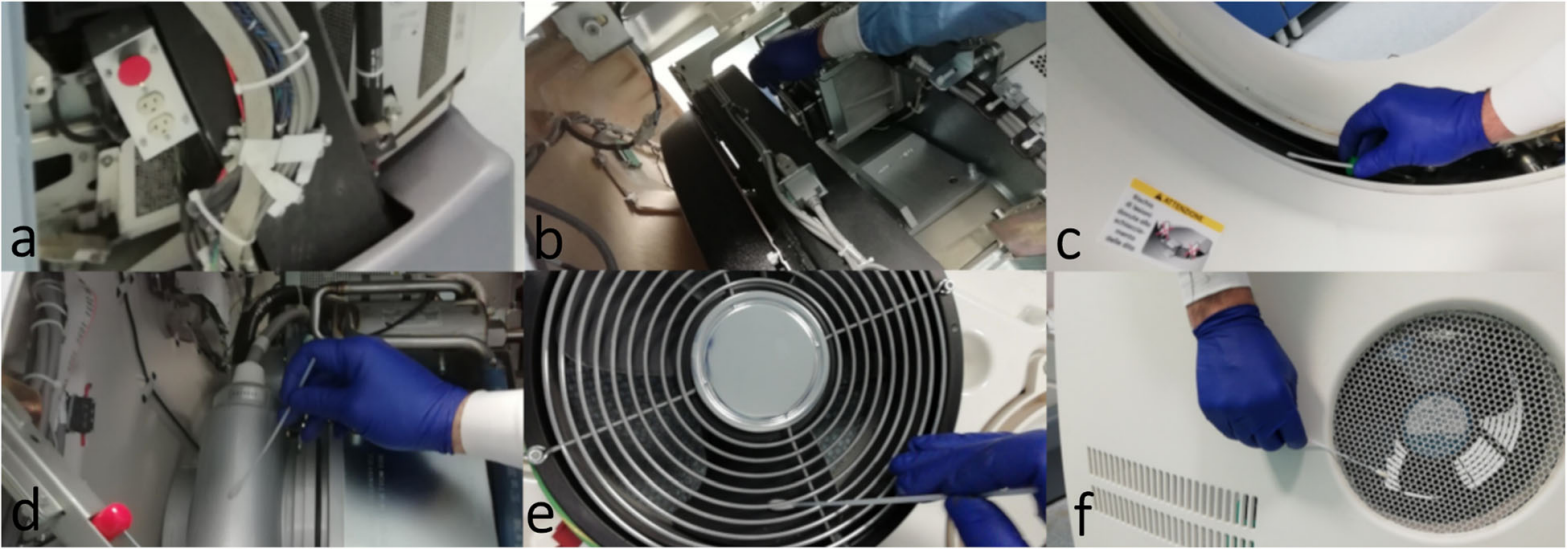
Computed tomography gantry internal component sampling. a Gantry case. b Inward airflow filter. c Gantry motor. d x-ray tube. e Outflow fan. f Fan grid
